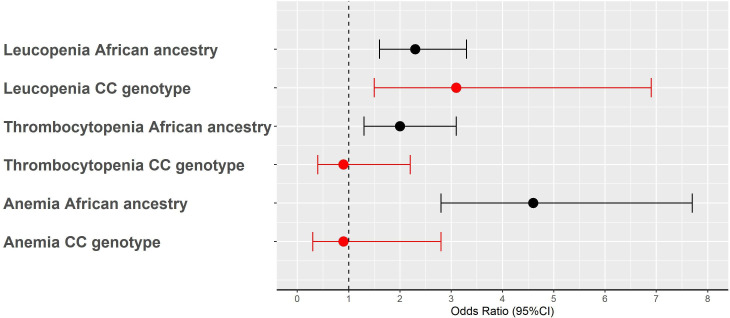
Risk of leucopenia, thrombocytopenia and anaemia in patients with SLE of African ancestry (AA) compared with European ancestry; and in carriers of rs2814778-CC genotype compared with non-carriers (CT/TT) among patients with SLE of AA. Black and red-filled dots and error bars represent the associations by ancestry and by rs2814778-CC genotype, respectively. Analyses were adjusted by sex, age, age at SLE diagnosis, length of follow-up after diagnosis of SLE and five principal components. Dots represent the OR and error bars the 95% CI.
